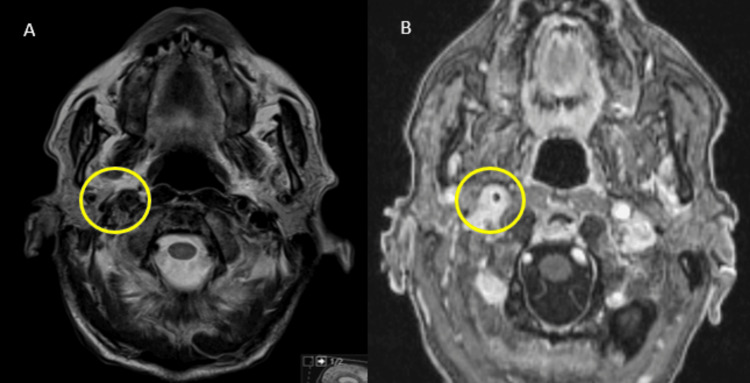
Axial T2 (A) and T1FS Gd (B) MRI denotes hypercellular infiltration with avid enhancement (B) of the right carotid sheath (yellow circles), findings similar to those in the thoracoabdominal aorta MRI: magnetic resonance imaging; T1FS Gd: T1 fat-saturated post-gadolinium
